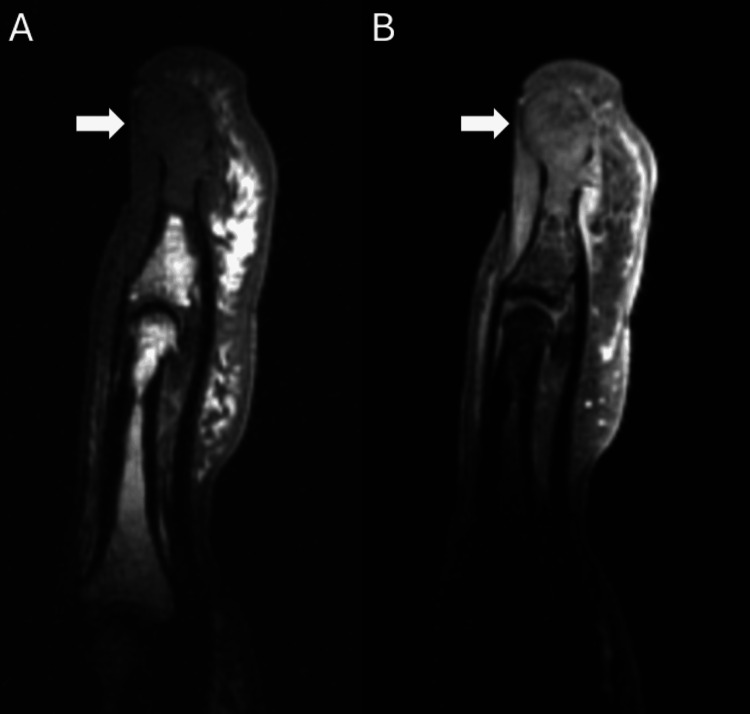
A) Sagittal T1 MRI of the left third distal phalanx, demonstrating an intraosseous mass of the distal phalanx that does not extend into the joint space. B) Sagittal T1 fat sat post-contrast MRI of the left third distal phalanx, demonstrating a solid enhancing lesion.
